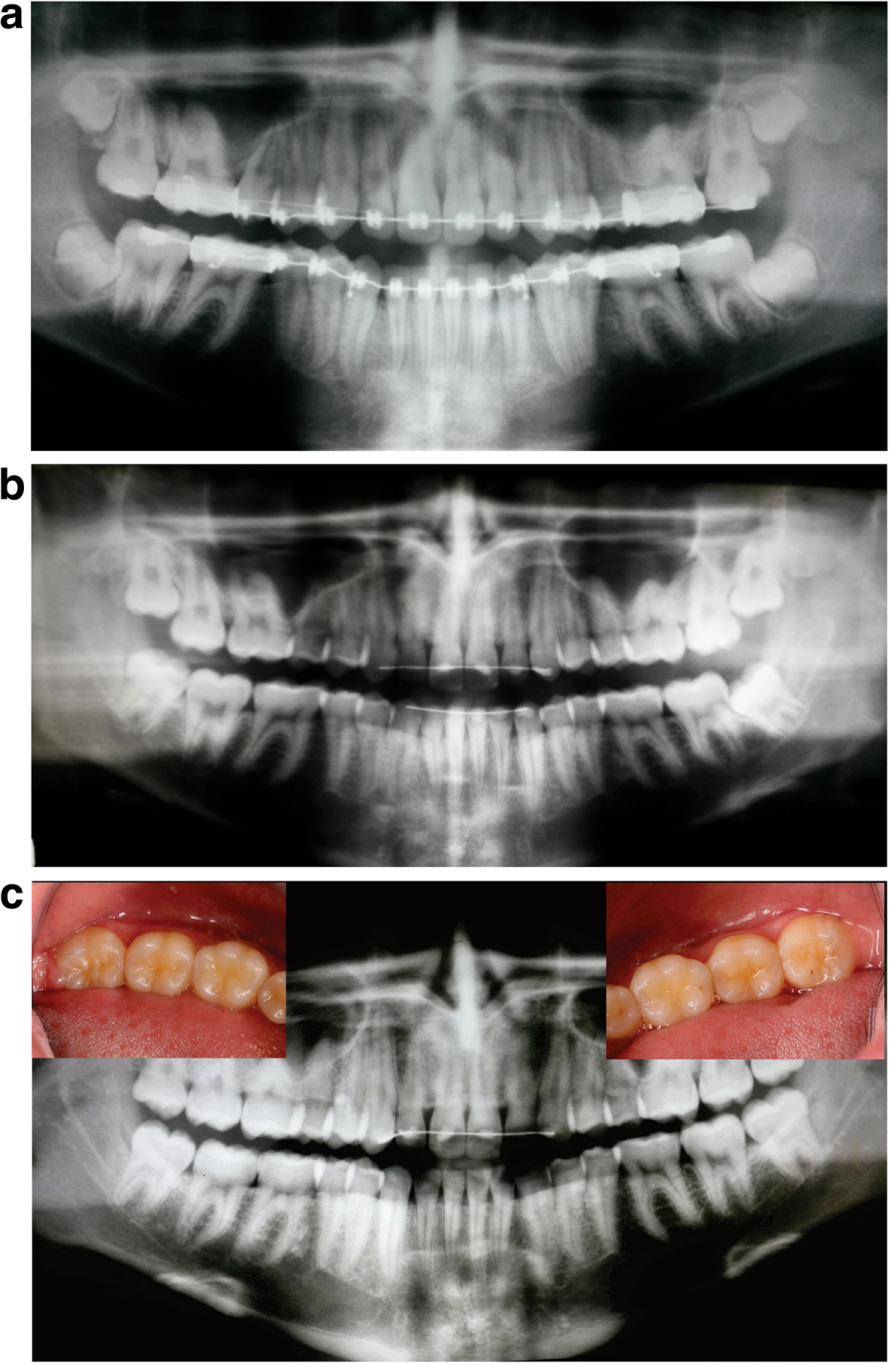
Panoramic radiographs of patient G just before bracket removal (T1, 14 years—a), 4 years after orthodontic treatment (18 years—b) and after complete eruption of the lower third molars (T2, 22 years—c). For this patient, OMFSs indicated third molar extraction in 82 % for the right side and 79 % for the left side, while orthodontists suggested 65 and 62 %, respectively
